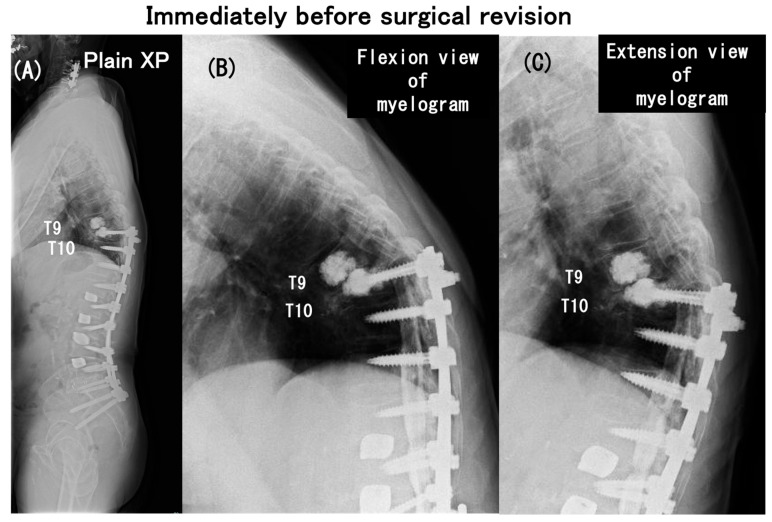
Plain radiograph (A) indicating PJK with segmental instability at the T9-T10 level (A) and lateral myelograms in flexion (B) and in extension (C) obtained immediately before surgical revision for paraparesis of modified Frankel grade D1, which developed as early as 6 months after the initial operation. The plain radiograph indicates PJK with segmental instability at the T9-T10 level (i.e., between UIV and UIV + 1) (A) and the lateral myelograms show a “block” to the flow of intrathecal contrast at the T9-T10 level with the T10 (i.e., UIV) fracture (B,C).
